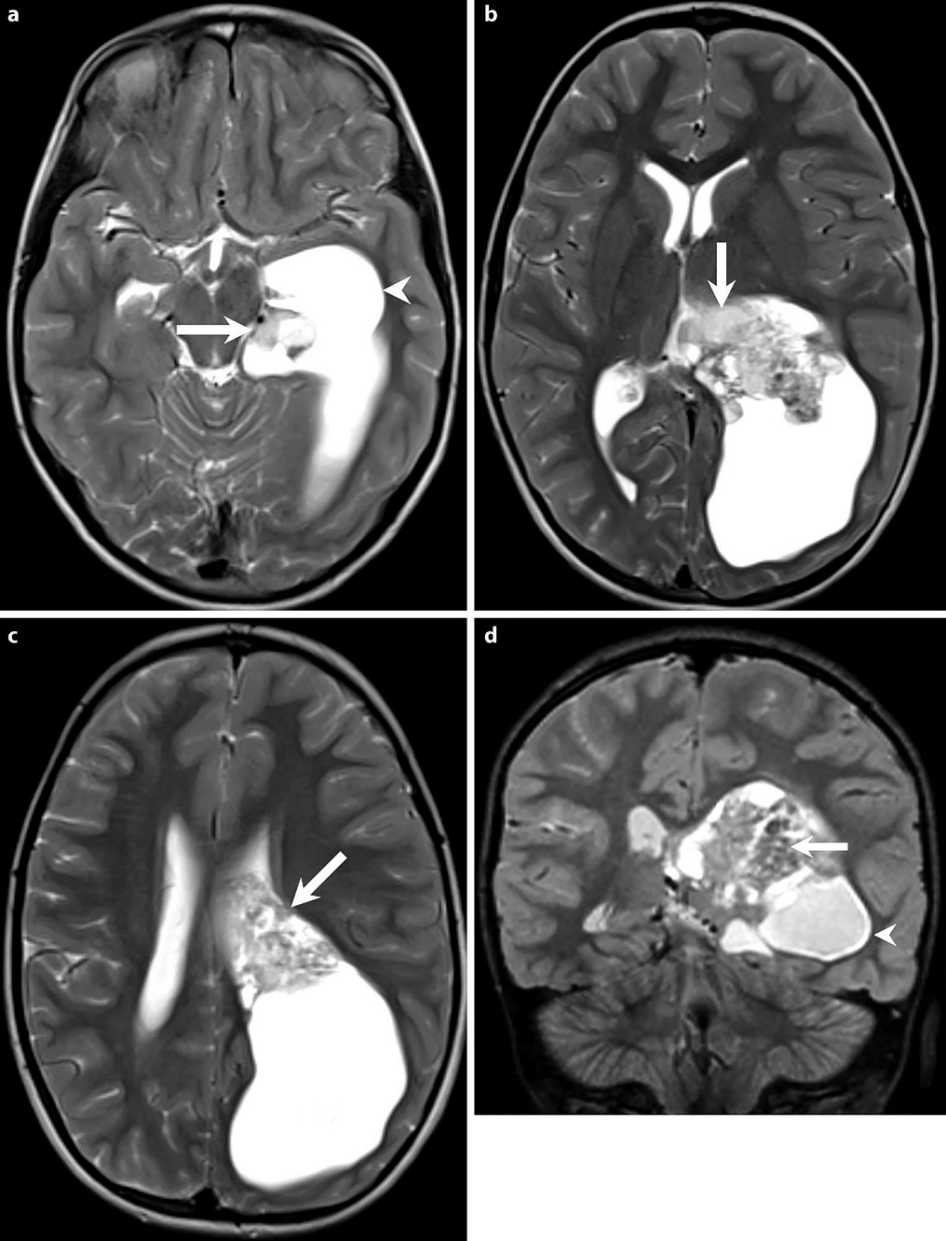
Axial (a–c) and coronal (d) T2-weighted images show an intraventricular tumor extending from the temporal horn through to the cella media of the left lateral ventricle (a–d, *arrow*). The tumor presents heterogeneous signal intensities with isointense portions next to smaller cystic components. The tumor leads to subsequent dilatation of the left lateral ventricle (a, d, *arrow head*)
